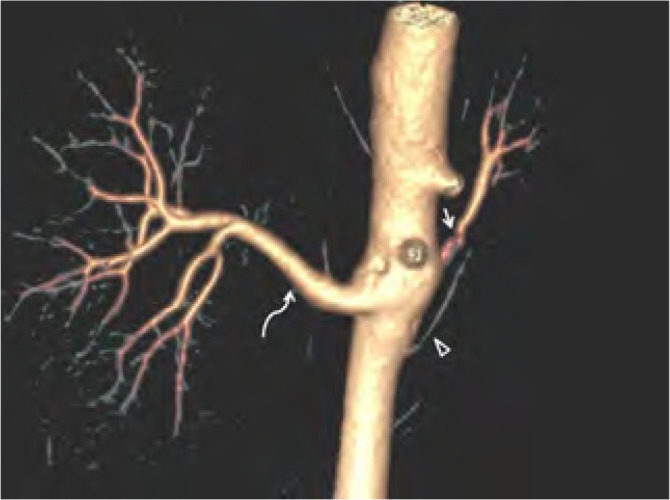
Volume rendered coronal image from CT Angiography depicts stenosis in the main renal artery (arrow), patent aberrant renal artery (arrowhead) on left with normal calibre right renal artery (curved arrow).
